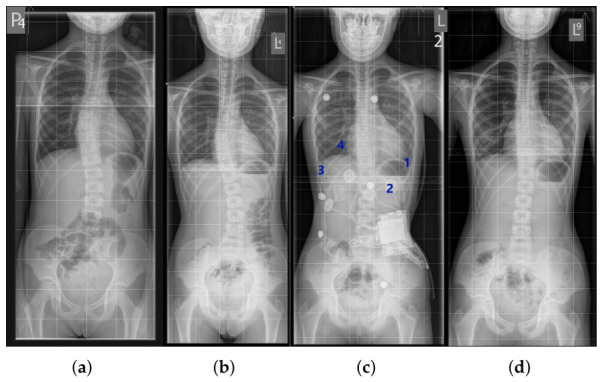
X-ray image of the second patient’s spine: (a) At the start of treatment. (b) During therapy. (c) In the orthopedic brace—full correction visible. (d) Without the brace—correction maintained.
